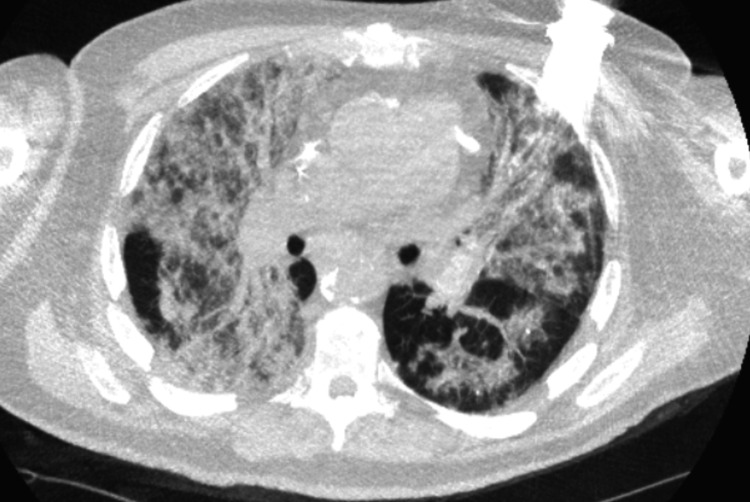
Chest CT demonstrating diffuse bilateral ground-glass opacities and interstitial changes consistent with immune checkpoint inhibitor–associated pneumonitis.
